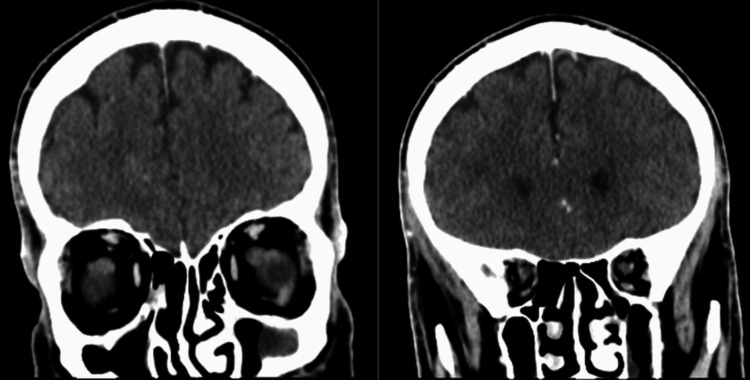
A contrasted CT scan of the paranasal sinus following completion of chemoradiotherapy showing complete resolution of the sinonasal mass.
